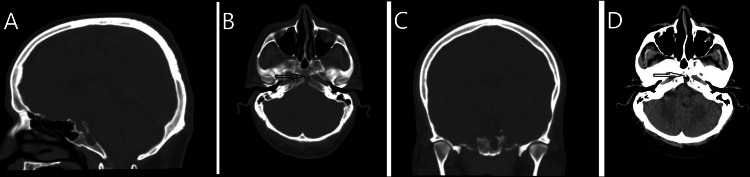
Non-enhanced brain CT Sagittal (A), axial (B), and coronal (C) CT scans of the brain on the bone window, along with an axial brain CT scan on the brain window (D), reveal a well-defined bony defect in the left side of the clivus. The margins of the defect are well-defined, smooth, and devoid of any signs of aggression. A subtle, tiny, stalklike hyperdense structure projecting from the clivus is also visible, which represents a pathognomonic osseous stalk (arrows on B and D). CT: computed tomography
